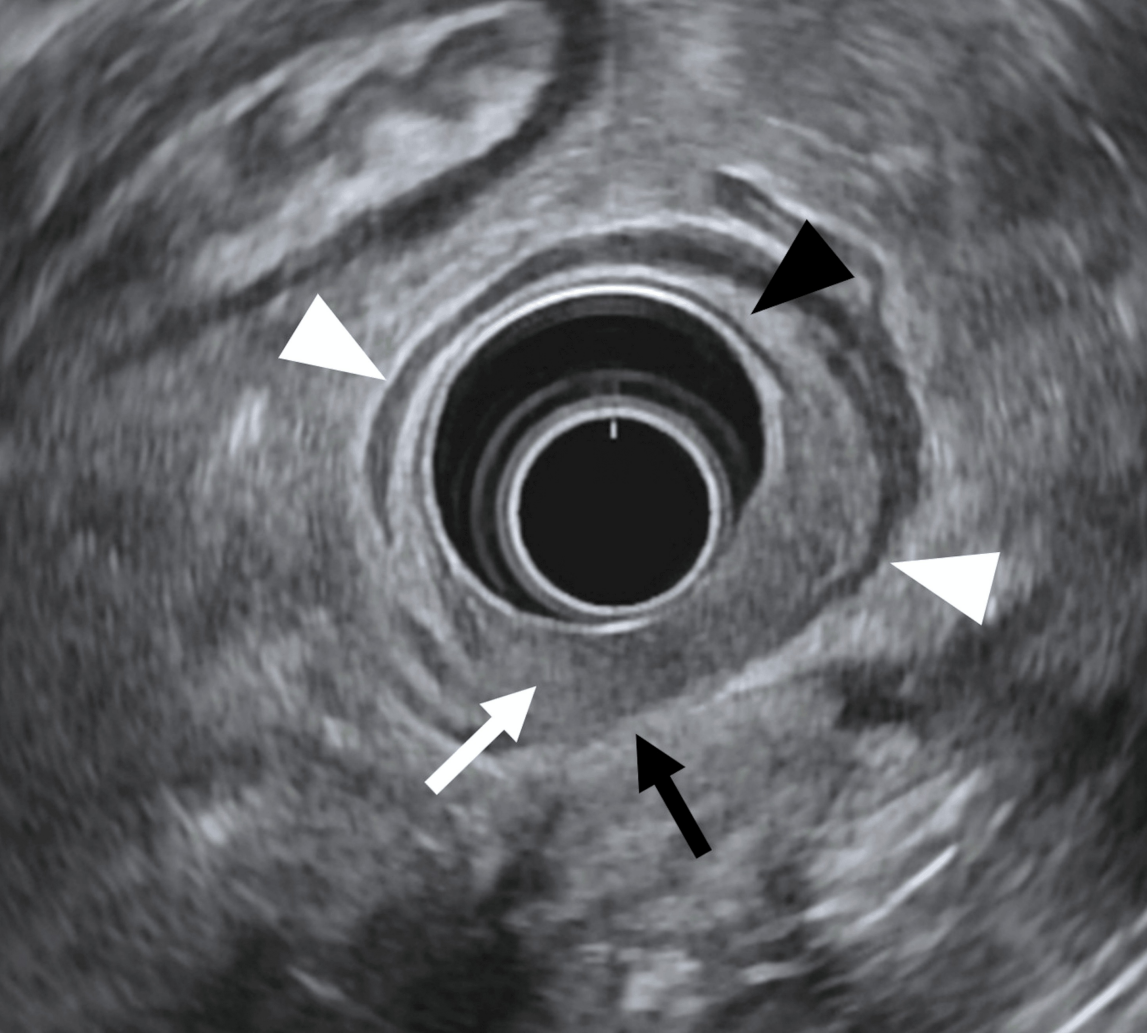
Endoscopic ultrasound of colonic mass with muscularis invasion. Endoscopic ultrasound shows a hypoechoic colonic mass (white arrow) extending into the muscularis propria. The normal hyperechoic submucosa (black arrowhead) is preserved anteriorly, while the muscularis propria (white arrowheads) is disrupted posteriorly (black arrow), consistent with tumor invasion.
